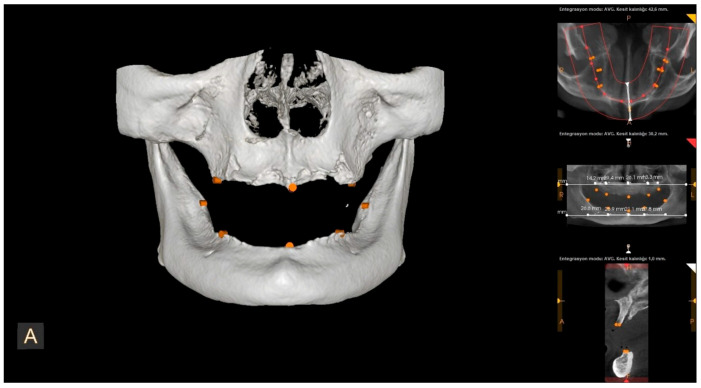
Landmarks on a CBCT image (front view).
